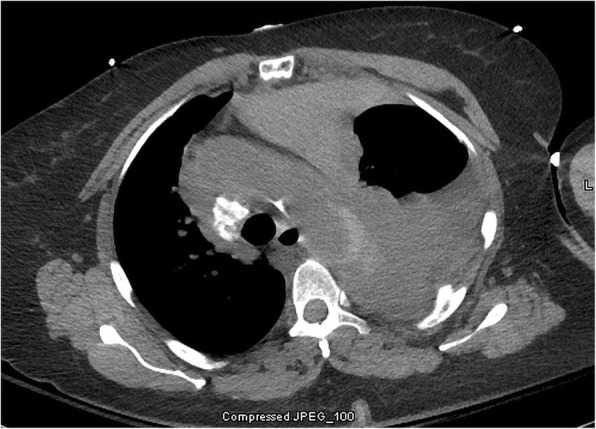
Pre-TEVAR CTA (axial view of non-contrast phase) showing crescentic aortic IMH originating in the distal aortic arch near the takeoff of the left subclavian artery and large left sided hemothorax
